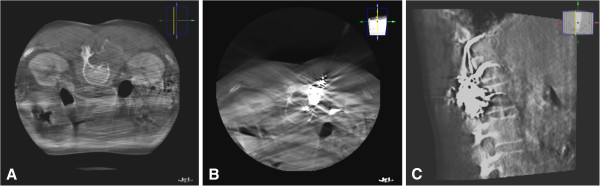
Planning CT scan (A) with contrast enhancement revealed a hyper vascular tumour formation at the right L1 level. Percutaneous injection of Onyx™ was performed (B) and intratumoural distribution was controlled by repeated CT scans (C).
